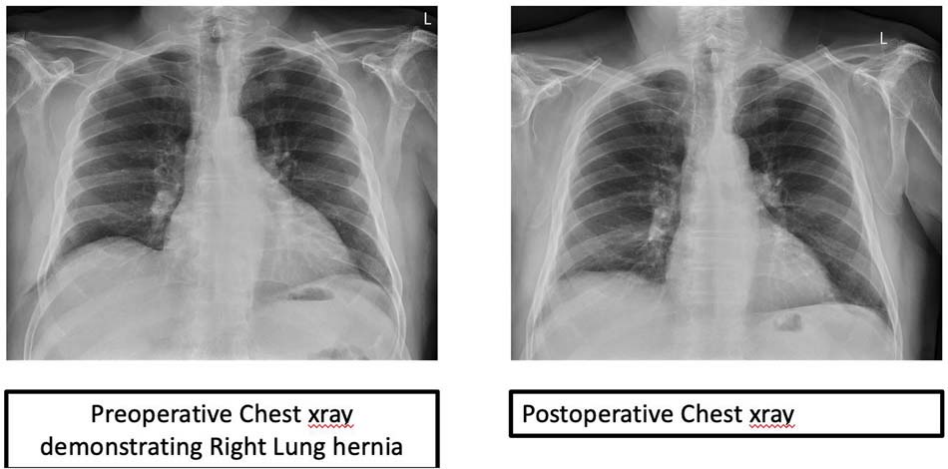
Preoperative and postoperative chest X-rays.
